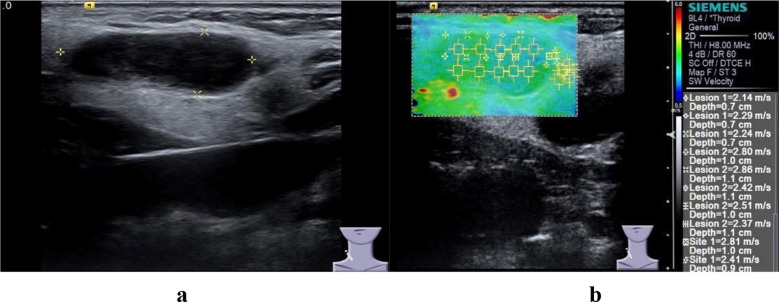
Benign cervical LN in a 34-year-old woman. a, Conventional ultrasonic images of malignant LN. b, Elastic ultrasound image of malignant LN.
